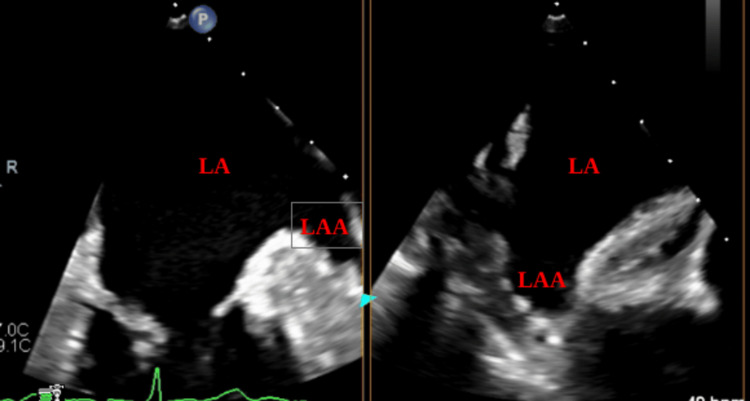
Transesophageal echocardiogram after six-week anticoagulation treatment showing complete resolution of the left atrial appendage thrombus with clear left atrial appendag (LAA) cavity.
